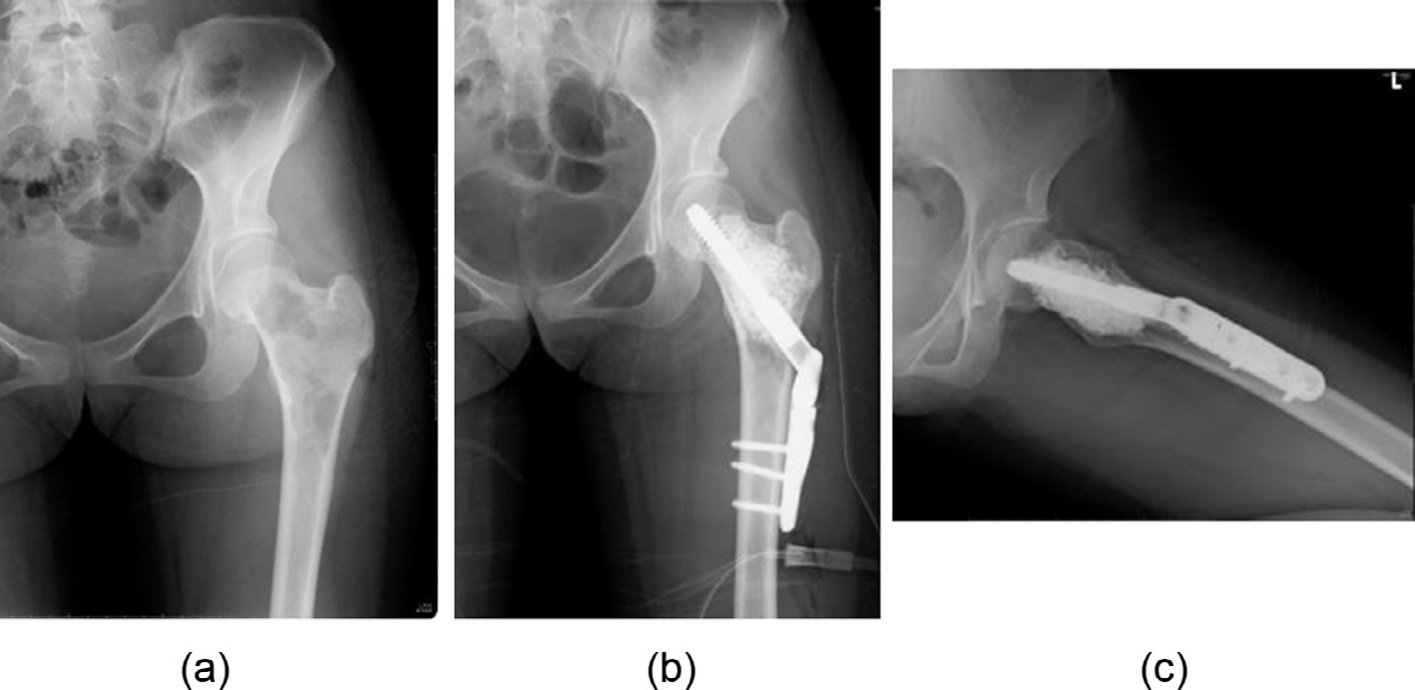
Radiograph showing fibrous dysplasia of the left proximal femur (a) (21-year-old female, Case 1). This patient with fibrous dysplasia was treated in combination with curettage of the bone tumor, the reinforcement using compression hip screw and implantation of CHA into the bone defect (b, c).
